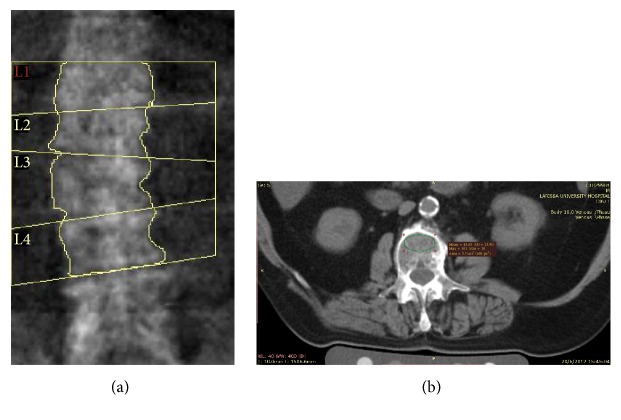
(a) DXA image of a lumbar spine with mild scoliosis in a male patient with COPD. DXA measurement of the L2 vertebra was consistent with normal BMD (1.054 g/cm^2^, *T*-score −0.4). (b) QCT measurement of L2 vertebra (56.3 mg/cm^3^) was consistent with osteoporosis. The patient also had a vertebral wedge deformity at T12. Notable abdominal aortic calcifications are noted in axial CT image that may have contributed to the discrepancy of the results.
